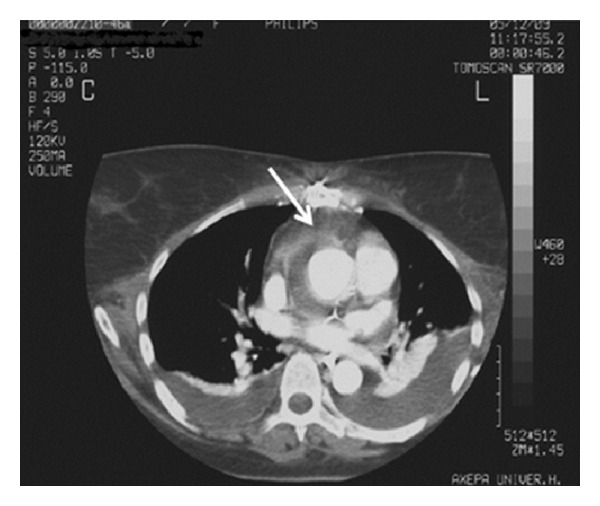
Thoracic CT with mediastinitis.
